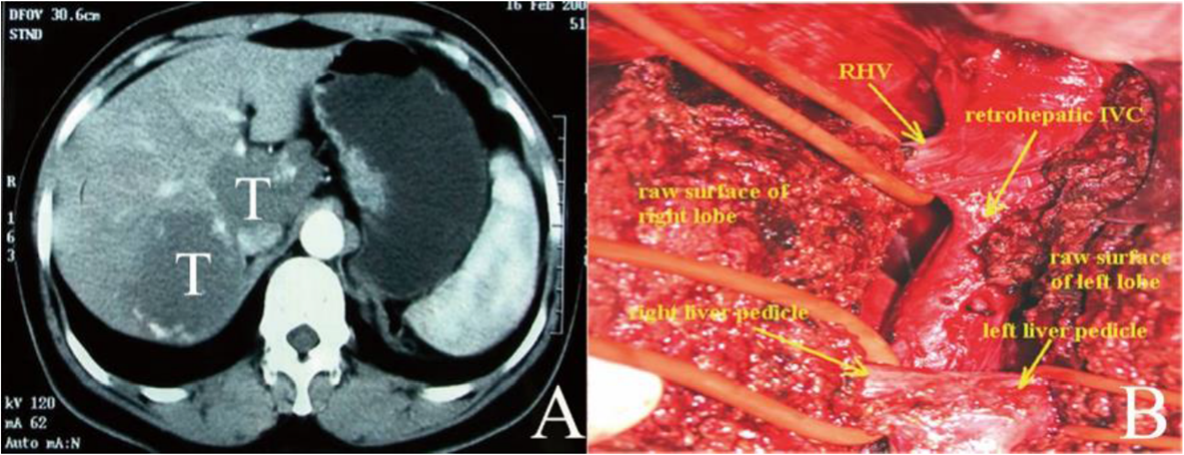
Complete resection of caudate lobe combine with S6 and S7 through anterior transhepatic approach (CT scan showed hemangioma in the whole caudate lobe and S6, 7. The intraoperative picture showed all the hemangioma removed)
